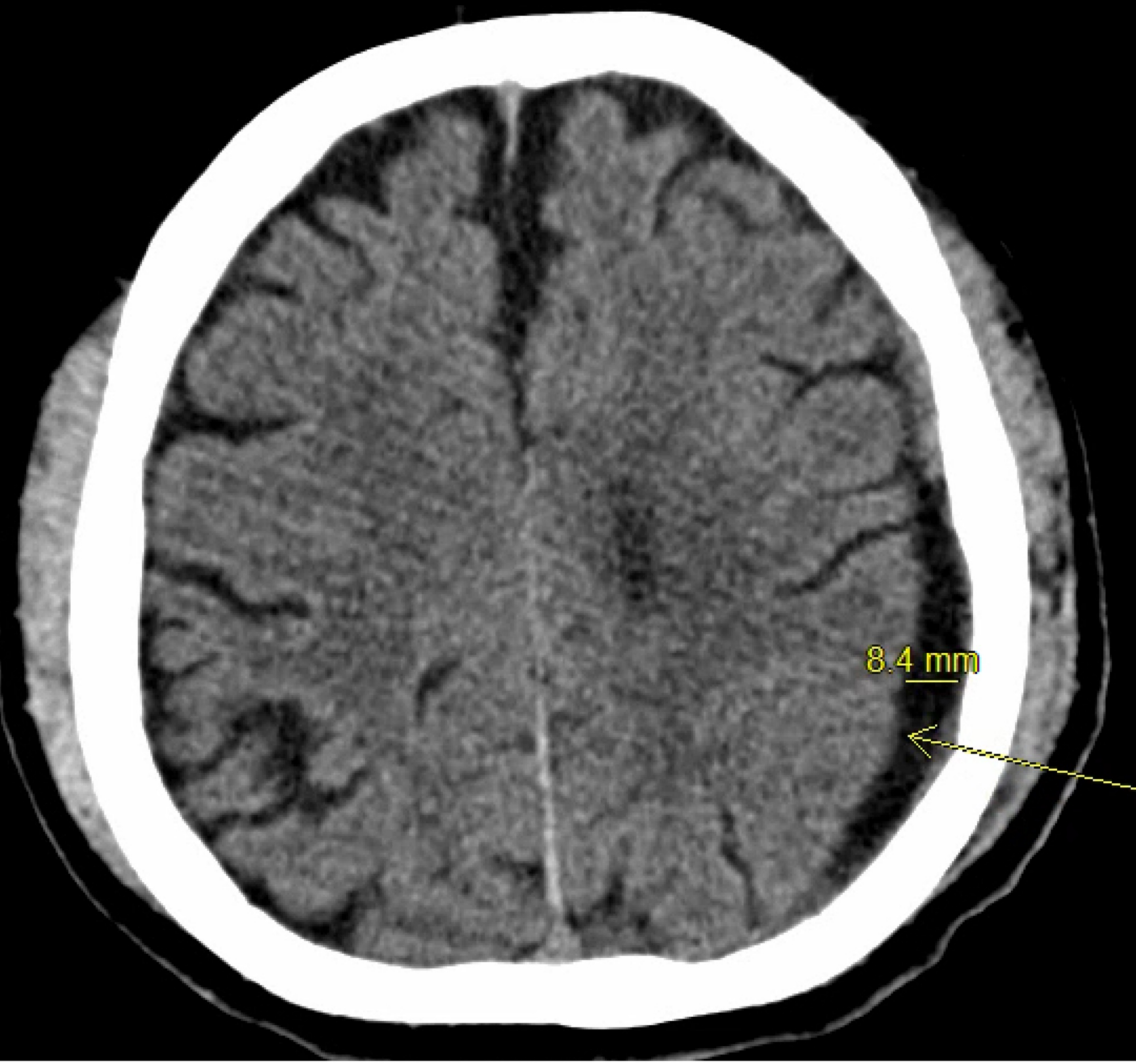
Post-tissue plasminogen activator and prior to discharge CT scan of a patient who underwent subdural drain placement with tissue plasminogen activator adjunct After the initiation of tissue plasminogen activator, the patient had increased drainage. This patient had significantly decreased mass effect and size of the subdural hematoma, marked by the arrow, after the initiation of tissue plasminogen activator protocol.
